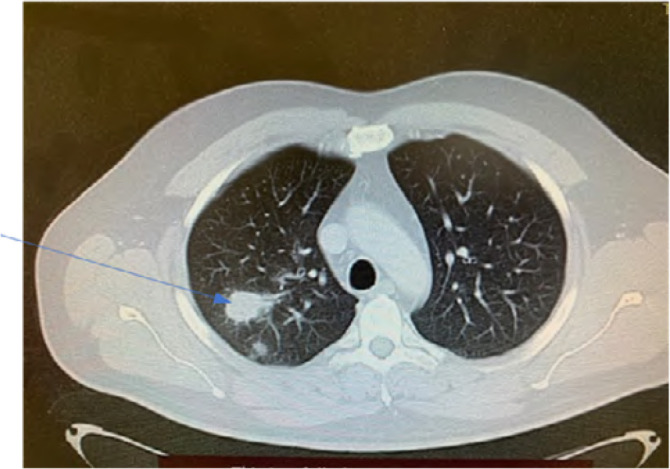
CT thorax with pulmonary granulomas.
